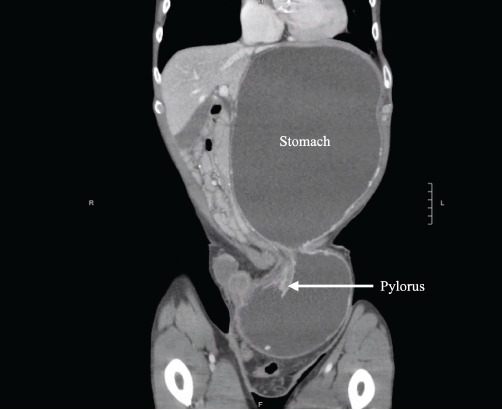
Computed tomography with coronal images demonstrating a stomach-containing inguinal hernia including the pylorus.
